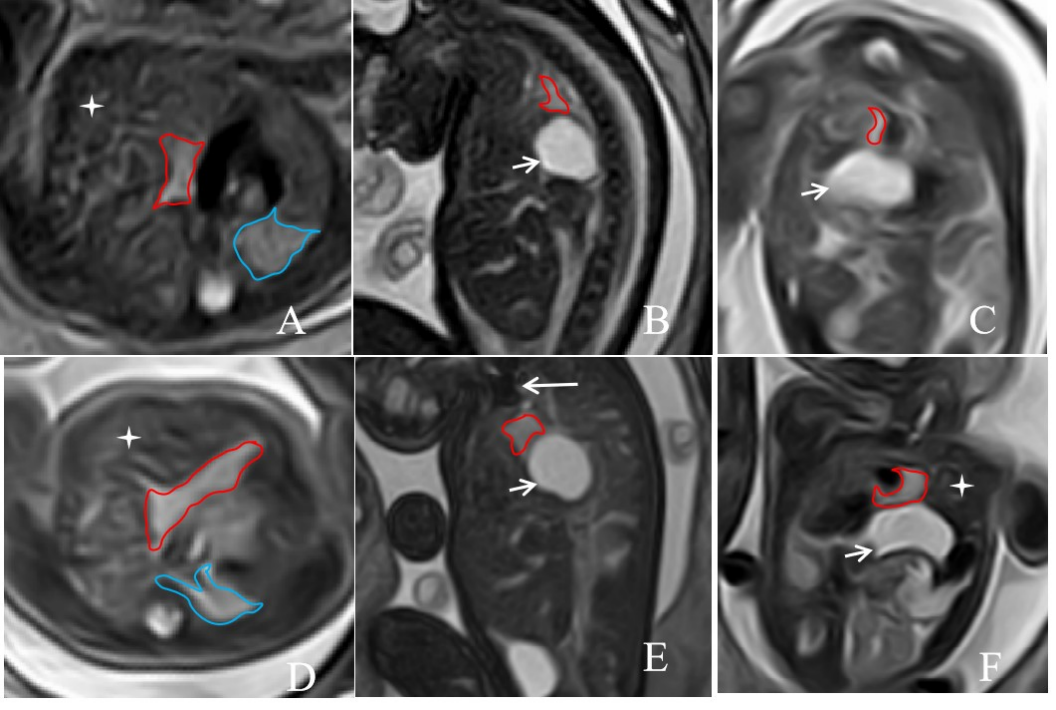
A 38-year-old pregnant woman, the fetus with left CHD on prenatal MRI. The axial, sagittal, and coronal T2-weighted images at 28w0d of gestation(A, B and C) before FETO treatment, and at 33w5d of gestation after FETO treatment(D, E and F) showed abdominal organs, such as the stomach, intestines, and partial left lobe of liver, herniated into the chest cavity(short arrow and cross Star). A T2-weighted image on a sagittal plane showed that a low-signal balloon was inserted into the trachea above the carina during FETO surgery (E, long arrow). The area delineated by the red line represents the left lung that has herniated into the chest cavity, while the area outlined by the blue line is the normal right lung.
